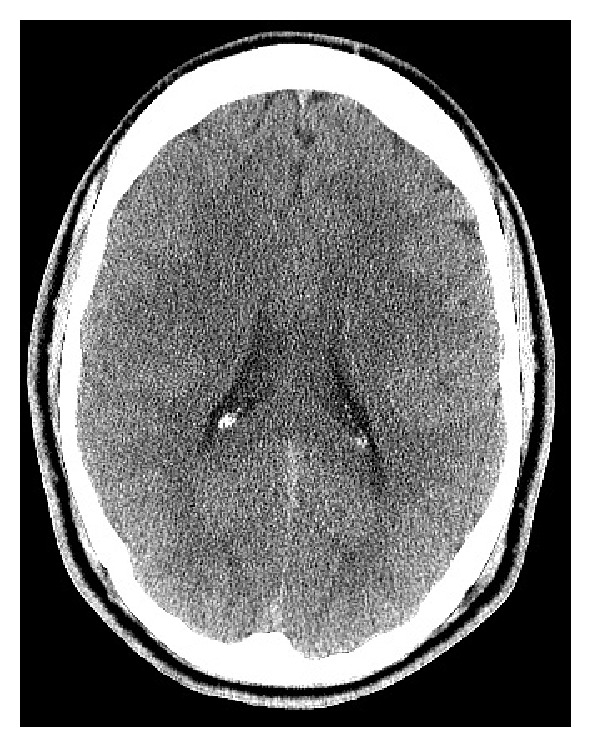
CT of the brain without contrast shows no intracranial abnormalities.
